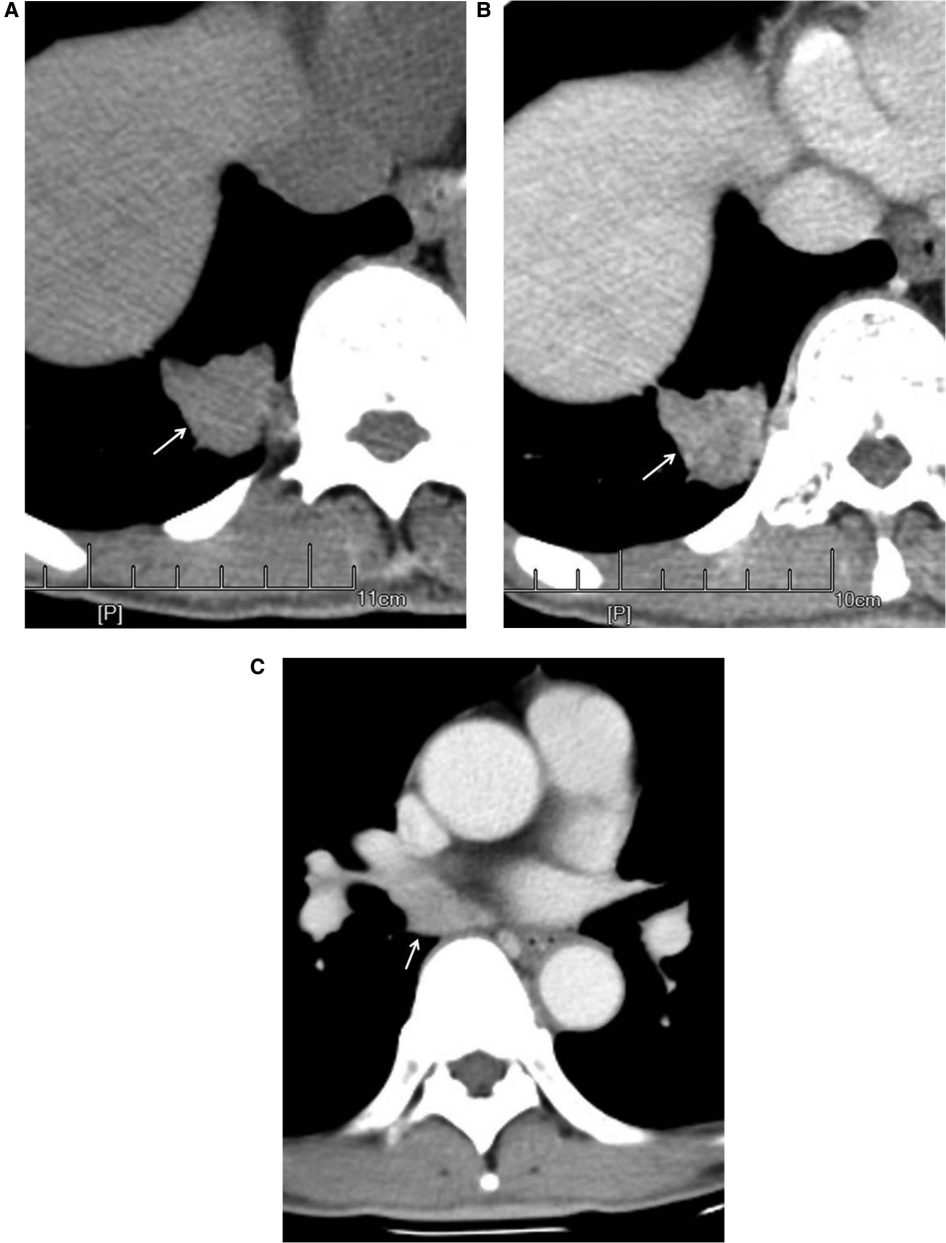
Male, 67 years old, with adenosquamous carcinoma in the medial basal segment of the lower right lung (moderately differentiated degree) with hilar and mediastinal lymph node metastases. CT showed a solid nodule. The left figure (A) shows unenhanced imaging and a solid nodule (arrow). The right figure (B) shows enhanced imaging and a heterogeneously enhanced nodule (arrow). Figure (C) shows lymphadenectasis in the right lung hilum and subtuberances (arrow) that were lymphatic metastases proven by pathology.
